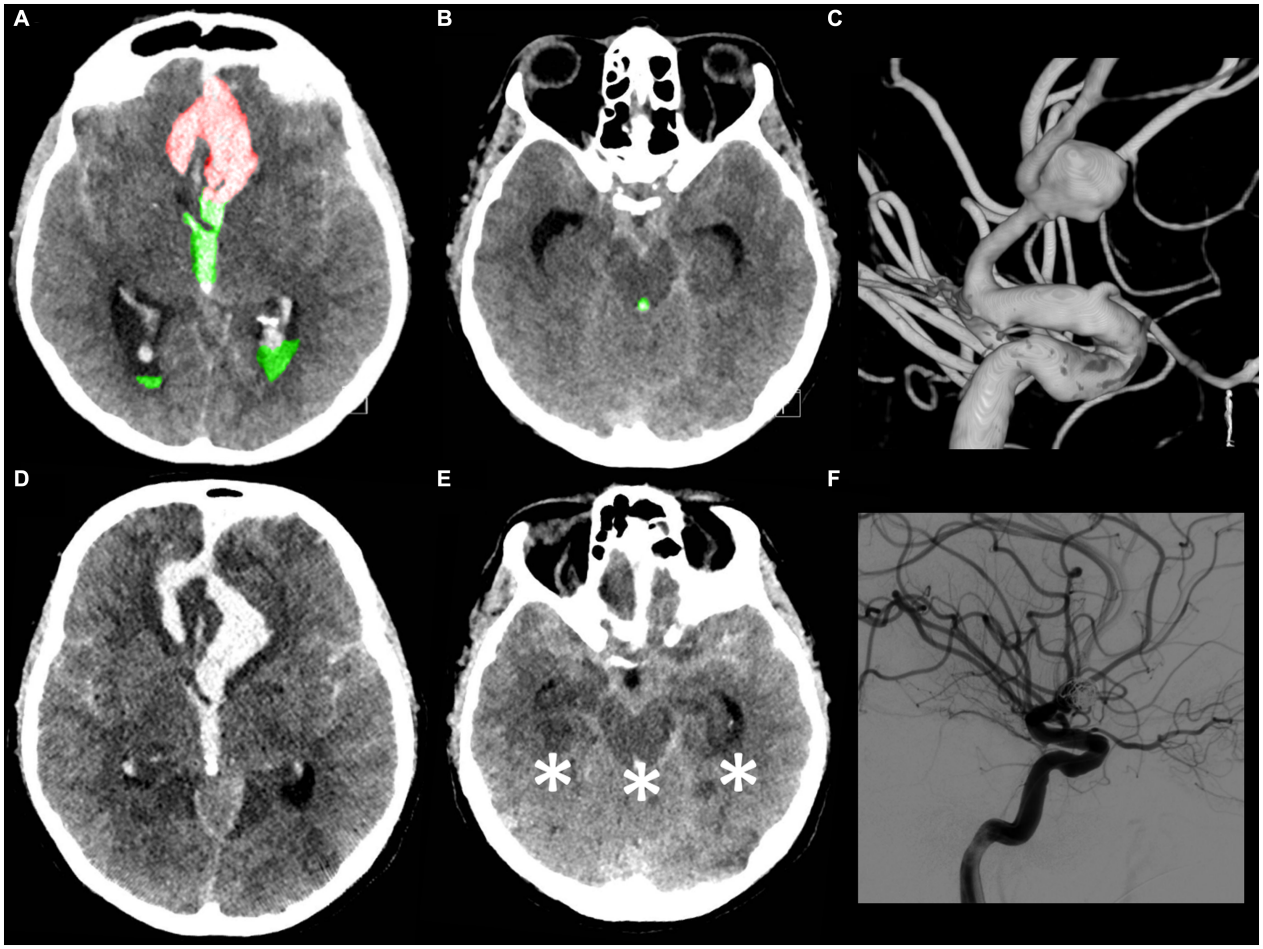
A 53-year-old male patient presented with a loss of consciousness. The WFNS score evaluation on admission was V with isochoric pupils and an intact pupillary light reflex. The initial CT scan (A,B) demonstrated the presence of a mFisher grade 4 aSAH with intraventricular hemorrhage (green A,B), volume 30 mL, and acute hydrocephalus with intraparenchymal hemorrhage (red A), volume 7 mL (IVH ratio 81%). The CT angiography and digital subtraction angiography (C) showed a ruptured communicating artery aneurysm. An urgent external ventricular drainage was placed and the aneurysm was endovascularly treated within 5 h of symptom onset. The 72 h post-treatment CT scan (D,E in corresponding slices as compared, respectively, with A,B) revealed the presence of bilateral temporal ischemia, occipital ischemia, and pontomesencephalic ischemia (asterisk E). (F) Post-coiling digital subtraction angiography showing aneurysm occlusion. The patient died 5 days after aSAH.
